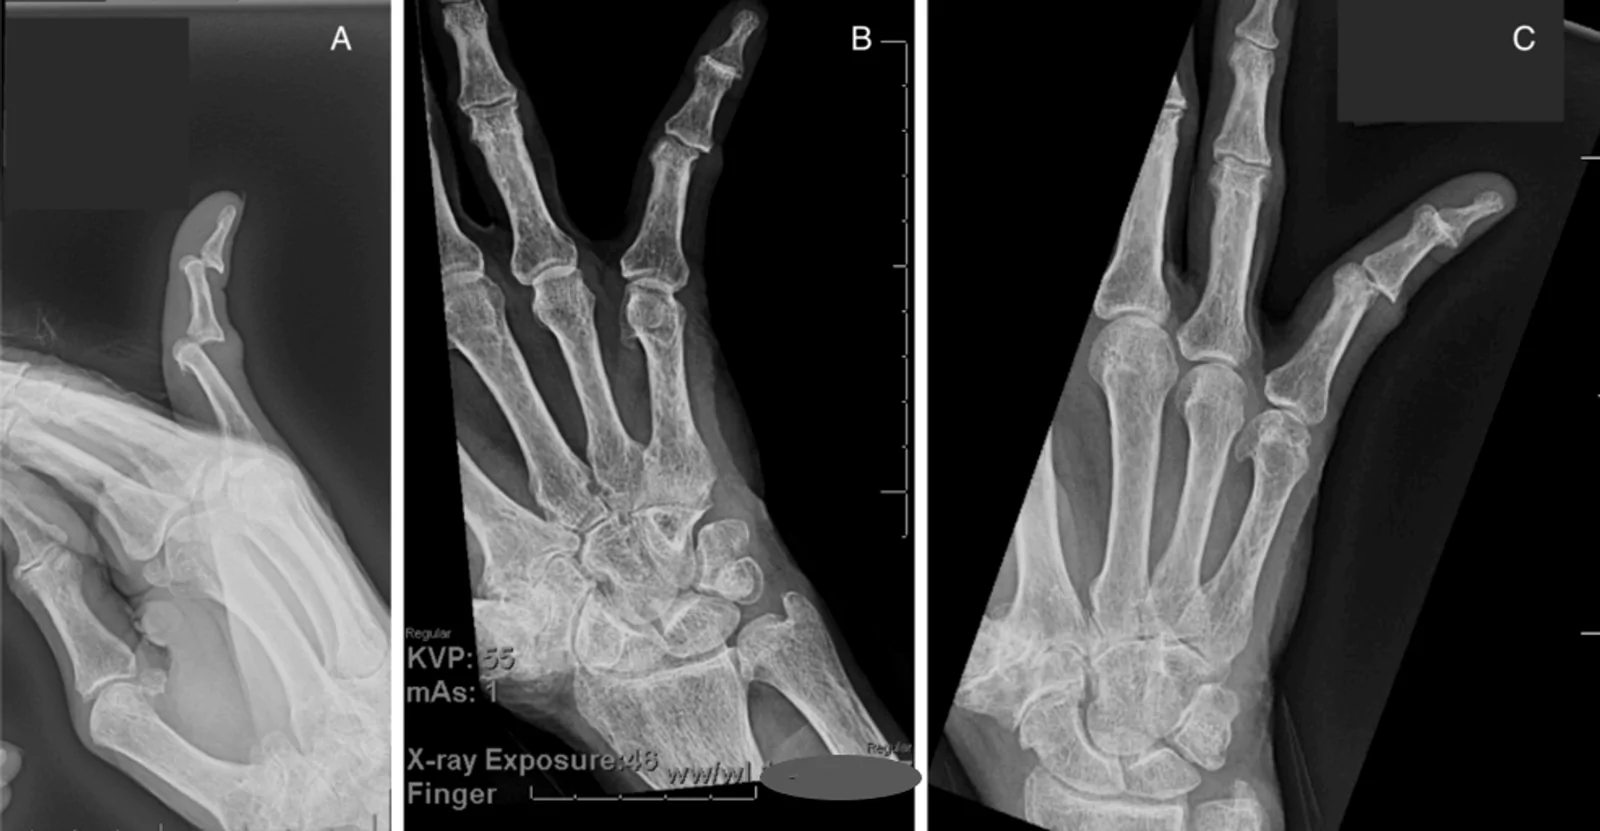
Lateral (A), anteroposterior (B), and oblique (C) radiographs demonstrating dorsal displacement at the PIP and DIP joints of the right fifth finger. PIP, proximal interphalangeal; DIP, distal interphalangeal
